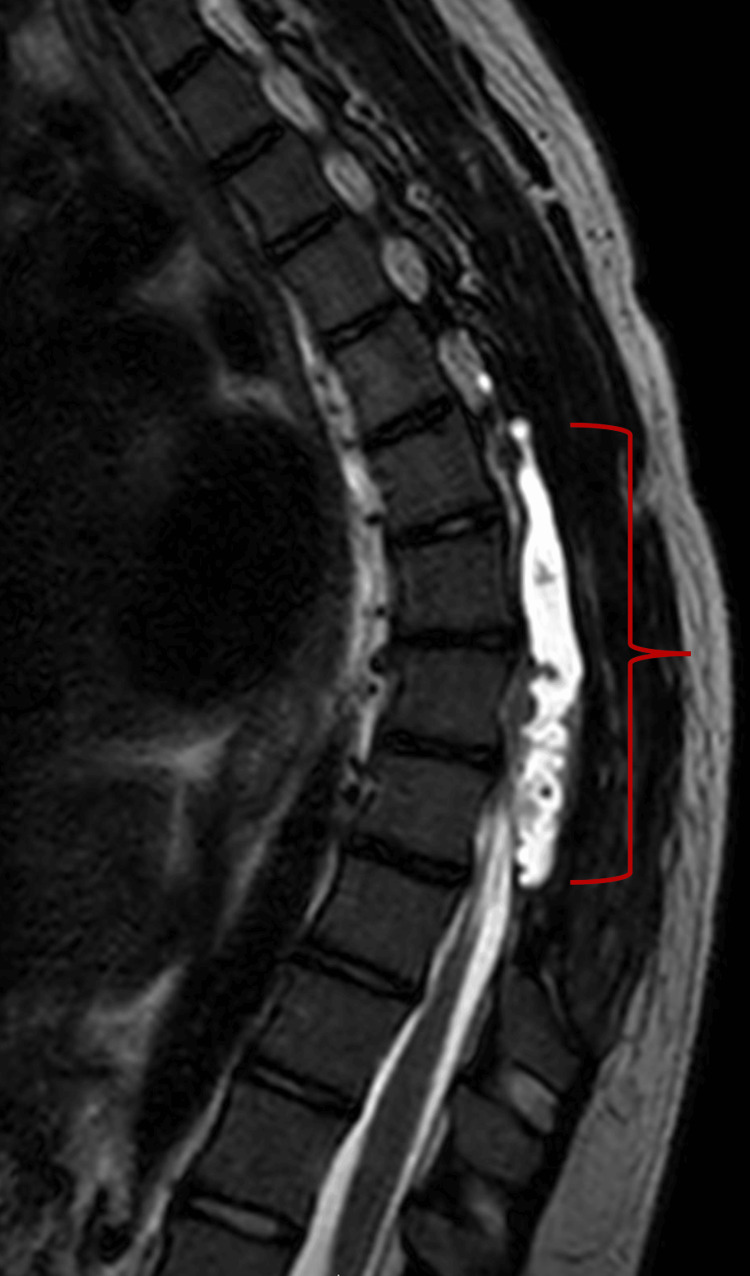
Sagittal T2-weighted MRI of the thoracic spine showing a posterior left dural defect with persistent spinal CSF collection and mass effect on the thoracic spinal cord.
